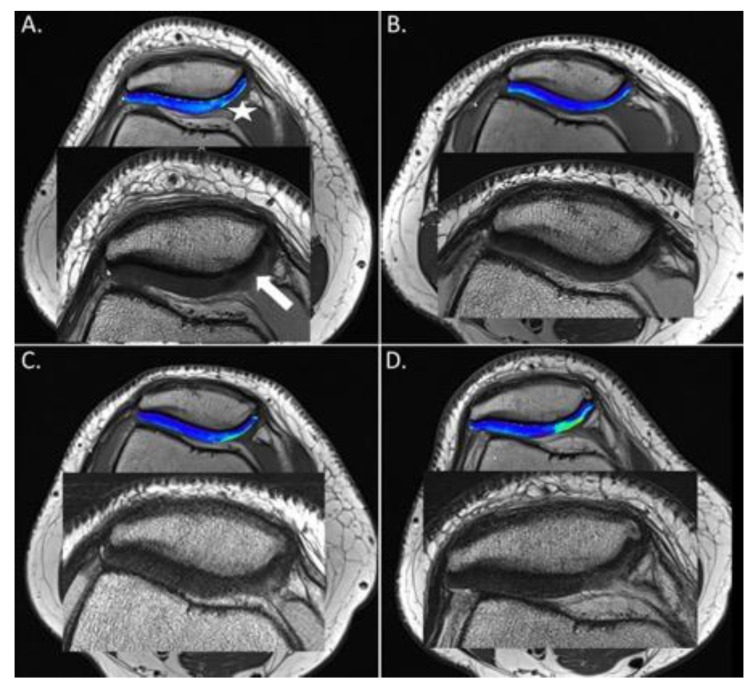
(A). Preoperative morphological magnetic resonance imaging (MRI) showing the International Cartilage Repair Society (ICRS) grade 2 cartilage defect at the medial facet of the patella (white arrow) and above the corresponding, color-coded T2 map. Note the increased T2 relaxation times of the defect, especially at the transition from the apex to the medial facet. (white star). (B) Two weeks postoperatively, the morphological MRI shows a full restoration of the cartilage surface including a hypointense area. The corresponding T2 map reveals a decrease in the T2 values compared to the preoperative status. (C) Four months and (D) twelve months postoperatively, the cartilage surfaces seem to break up and the corresponding T2 values increase significantly.
